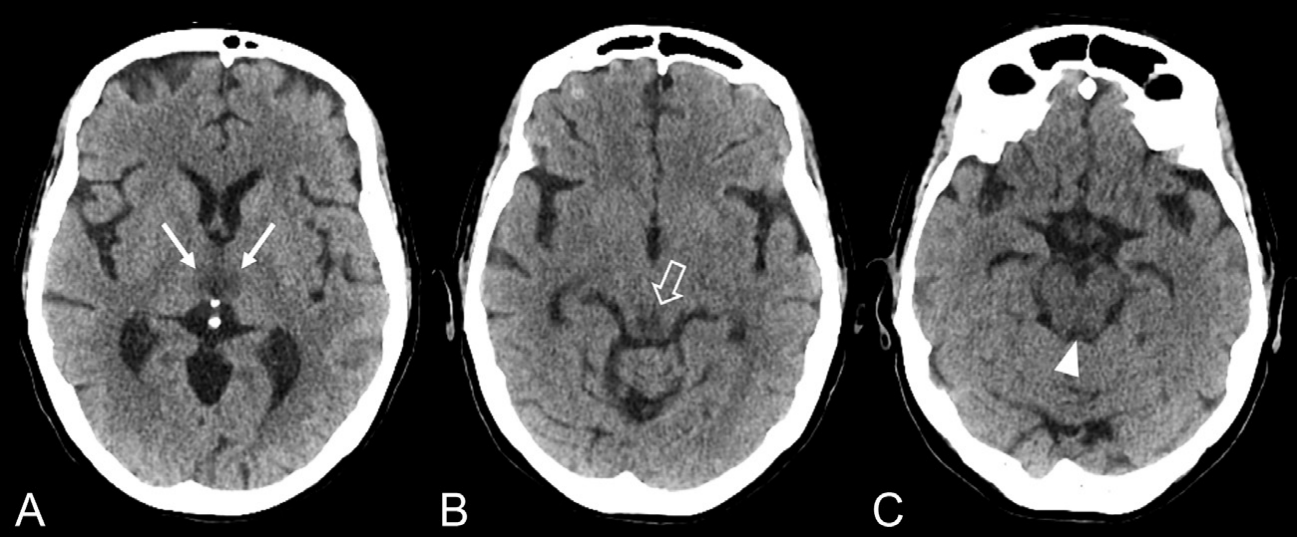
Unenhanced CT scan shows hypoattenuation in both medial thalami (thin arrow in A), the tectal plate (arrow in B) and periaqueductal region (arrowhead in C).
